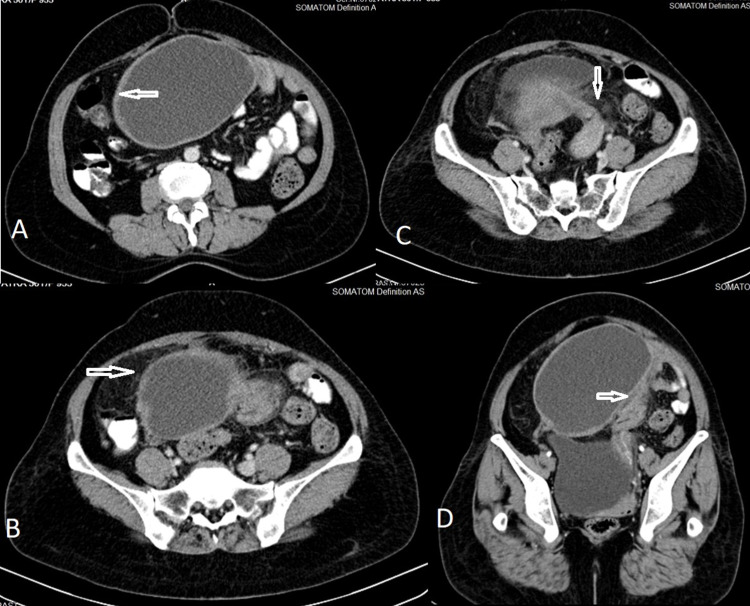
Case 2 CT axial sections (A, B, C) and oblique coronal reformation (D) showing a unilocular cystic lesion, anterosuperior to the uterus with thick walls (arrow in A), perilesional fat stranding (arrow in B), and twisted pedicle with ‘helical swirl’ appearance in continuous with left cornu of the uterus (arrows in C & D)
